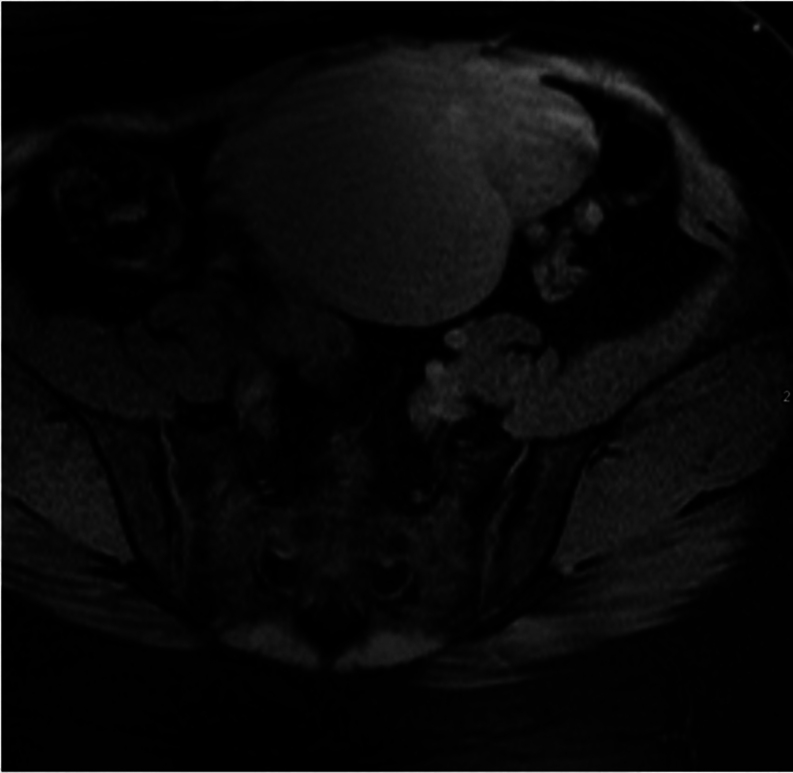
Pelvic MRI mass within the right pelvis that arose from the right adnexa (case 1).
